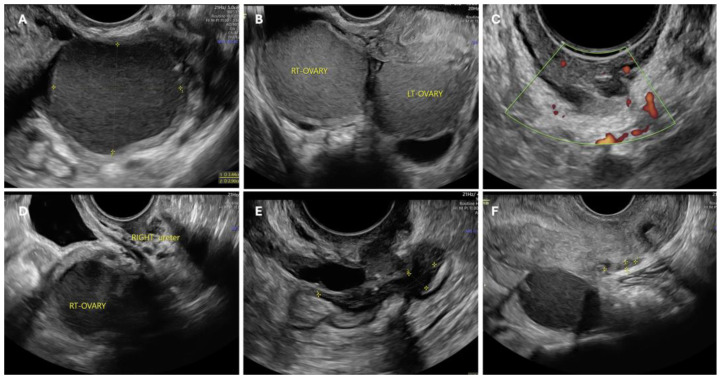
Different ultrasonographic cases of pelvic endometriosis. Ovarian involvement may be unilateral (A) or bilateral (B), giving the characteristic ‘kissing ovaries’ sign when the two affected ovaries are attached posteriorly to the uterus. Parametrial endometriosis, nodule involving the right ureter (C). It is important to note that pelvic structures are closely located and therefore it is common for adjacent structures to be infiltrated or retracted by endometriosis, as in the case of the ureter (D,F). Figure (E) shows a case of endometriosis of the anterior wall of the rectum. Often the lesions are multiple, resulting in complex presentations with possible involvement of the ovary, uterosacral ligaments, and rectum, resulting hypomobility of the structures, Douglas’ obliteration and even frozen pelvis.
